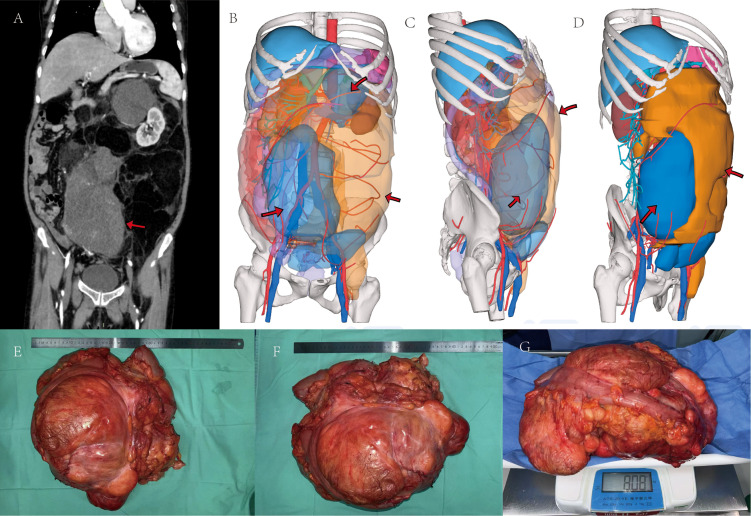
(A) Sagittal abdominal CT scan demonstrating a large, heterogeneous intra-abdominal mass. (B-D) Three-dimensional reconstructions highlighting solid (blue arrows) and fatty (yellow arrows) components. (E-G) Intraoperative view of the resected tumor, measuring approximately 40 × 45 cm and weighing 8.08 kg.
